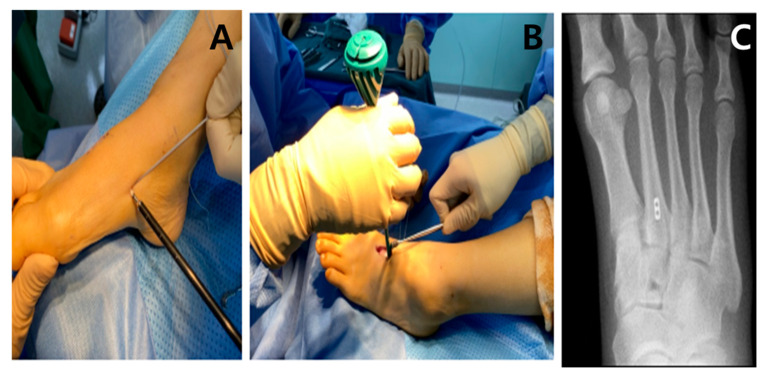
Surgical techniques for stabilization. (A,B) Internal Brace technique with suture tape and anchors providing flexible fixation across the joint. (C) Postoperative weight-bearing radiograph demonstrating that swiveLock anchor secures the FiberTape under tension, providing knotless fixation.
